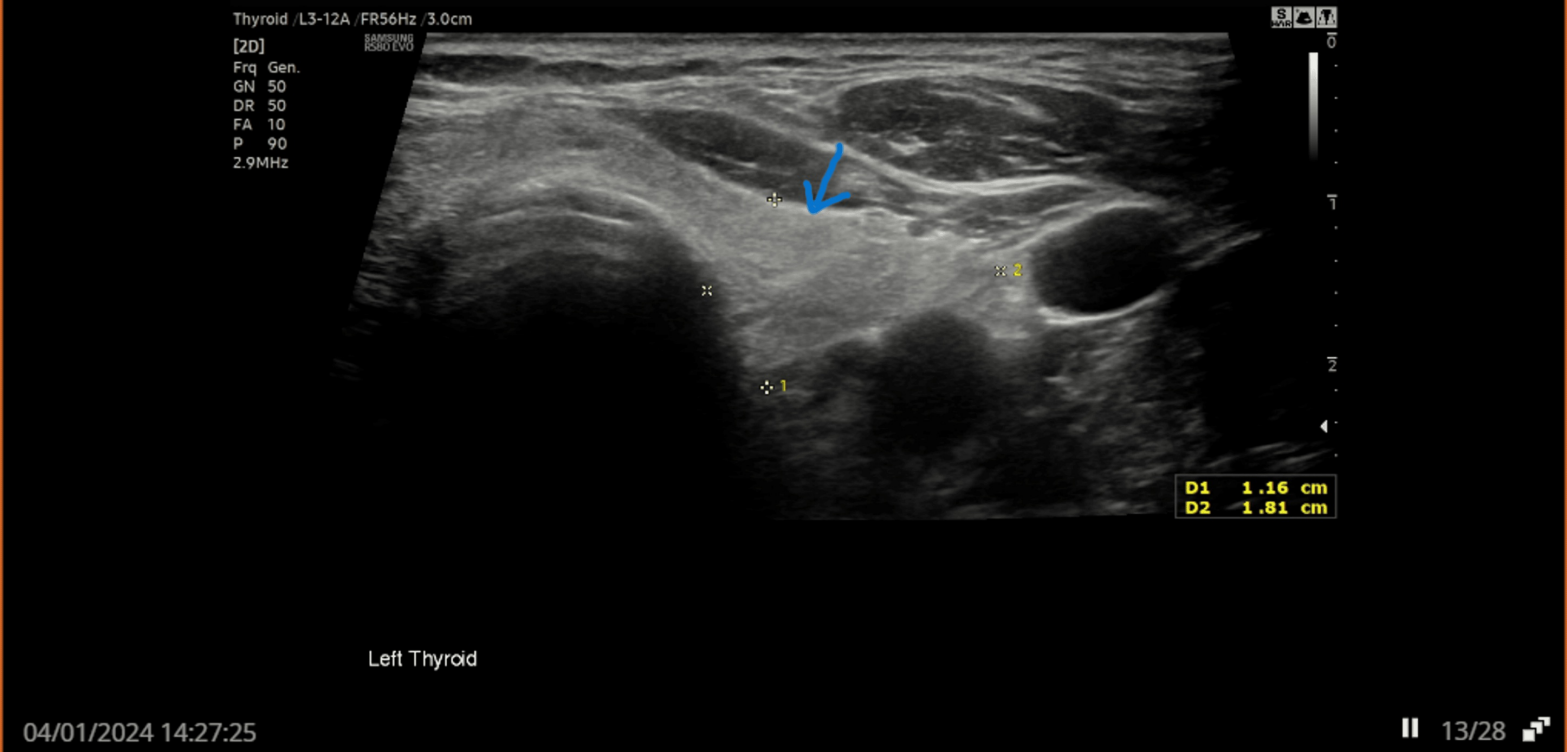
Ultrasound thyroid showing isoechoic U2 nodule in the left lobe Arrow showing isoechoic U2 nodule in left lobe of thyroid. U2: benign or non-cancerous nodule.
